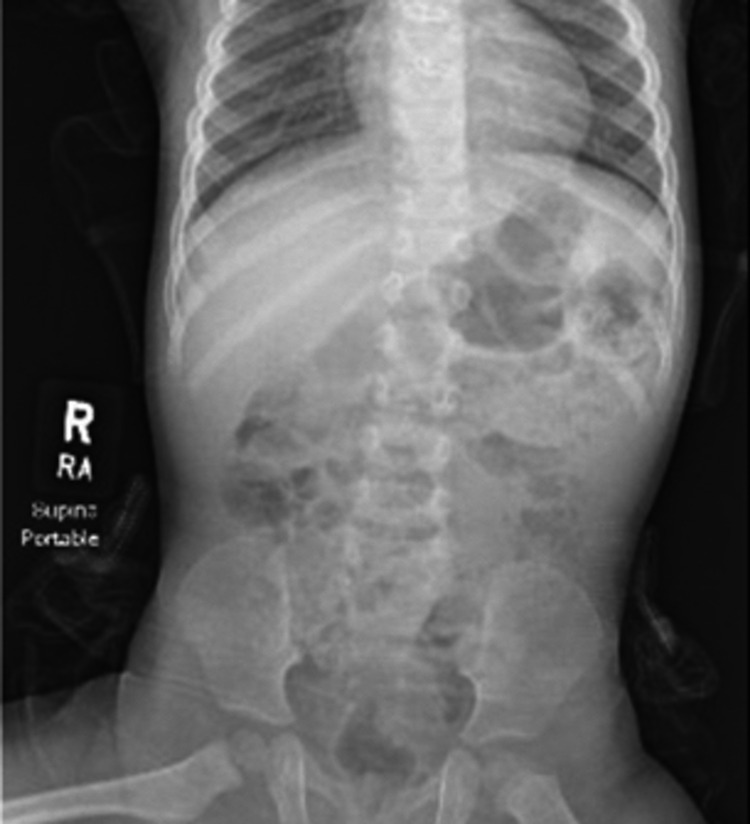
Single-view abdominal plain film showing distal bowel gas Single-view abdominal plain film showing normal caliber gas and stool in the proximal and distal bowel, with no evidence of obstruction. There is mild-to-moderate gaseous distention, without the classic double "bubble sign" of one gastric air bubble and one proximal duodenal air bubble, that would suggest duodenal atresia.
